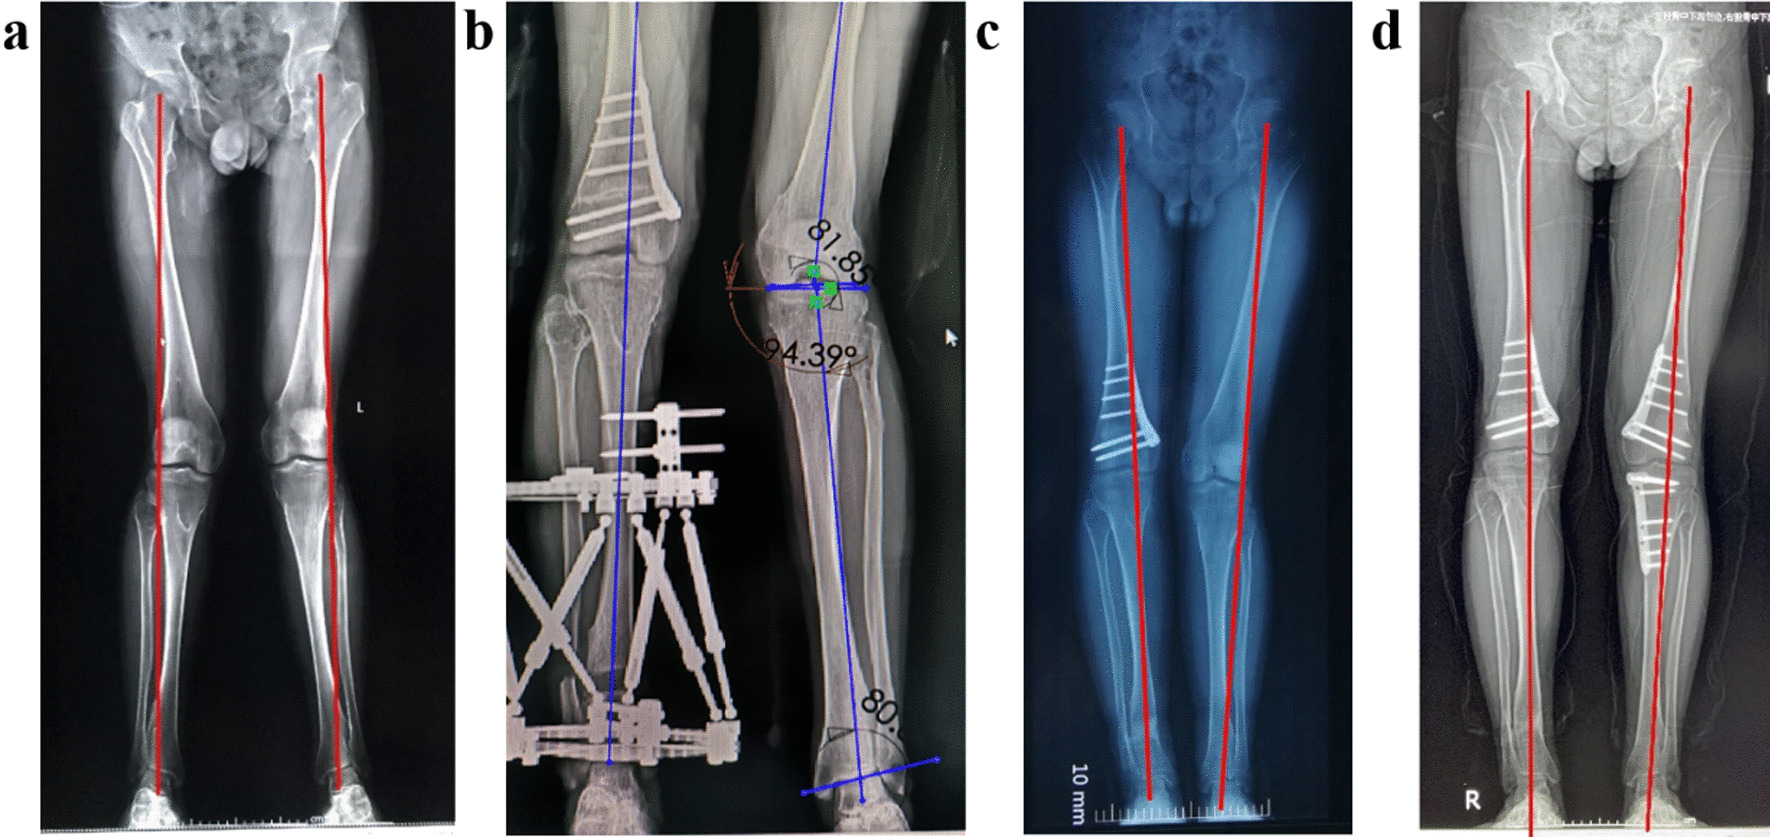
An 18-year-old patient had bilateral knee and malleolus valgus because of multiple osteochondroma. The right lower limb was 3.3 cm shorter than the left. a Full-length radiograph before operation. b X-ray after operation shows the deformity has been corrected. c Left lower limb surgery will be taken after 1 year. d Osteotomy and internal fixation at the left side. Re-examination X-ray shows the osteotomy site healed well. The lower limb alignment of right limb and the LLD is near normal
